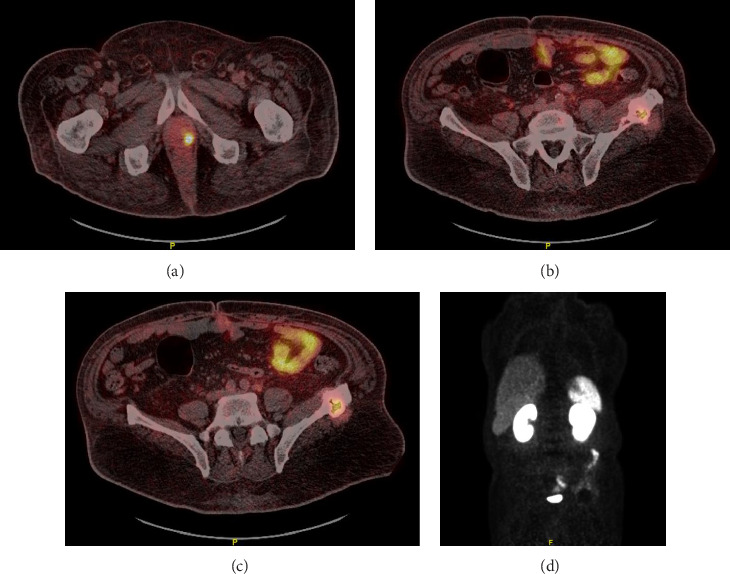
PSMA PET-CT imaging findings. (a) PSMA avid lesion in the posterior aspect of the left prostate lobe; (b–d) PSMA avid left retroperitoneal and left iliac chain nodes and PSMA avid lesion within the left iliac bone extending from the iliac wing down to the anterior acetabulum.
